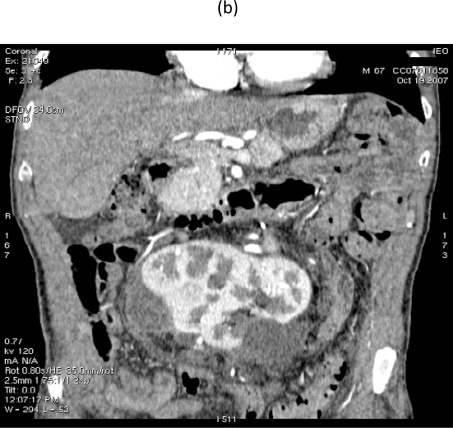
Supernumerary artery for the lower pole of the left kidney was sacrificed during surgery. Follow-up CT images show infarction of the left kidney upper lobe (a); however, the remaining parenchyma of the left kidney was well perfused (b)
